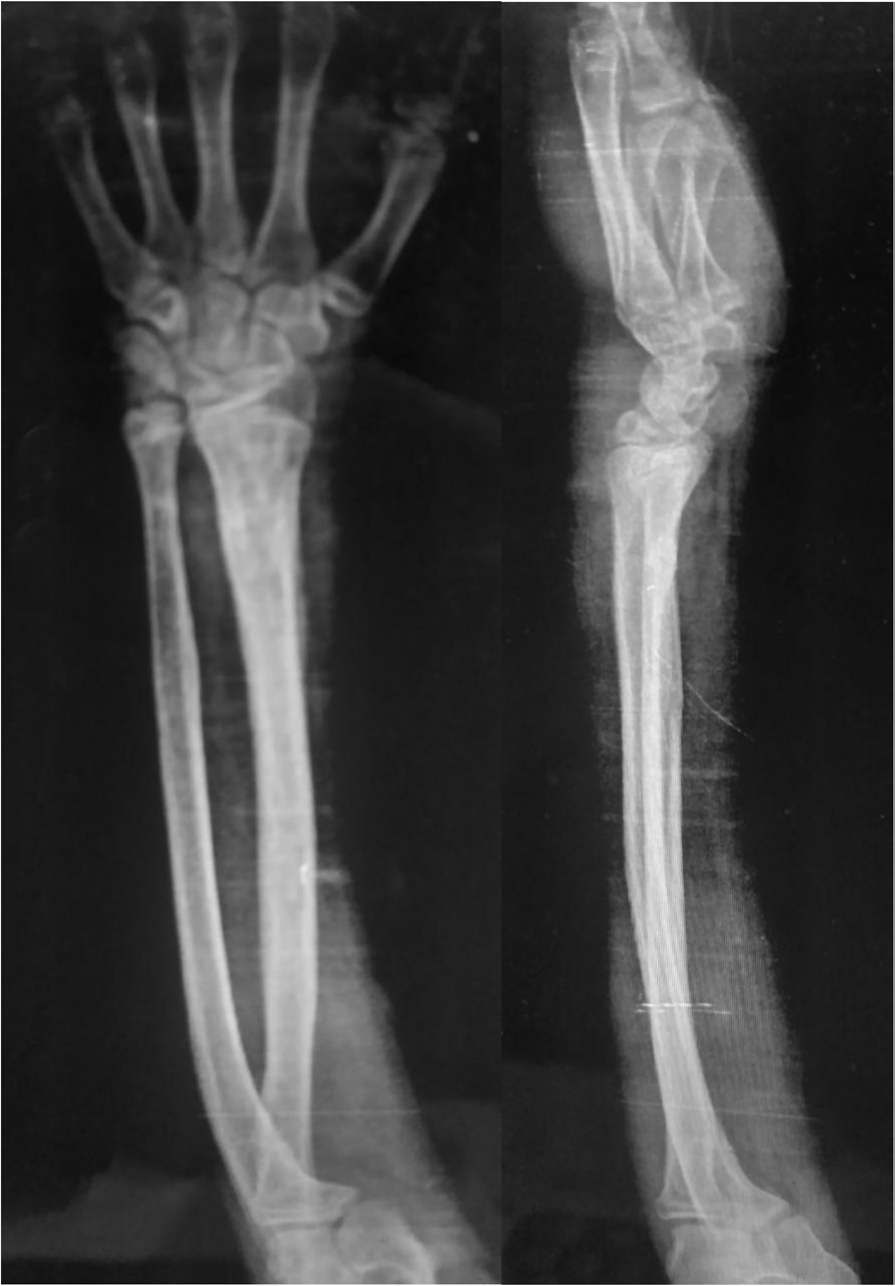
Anteroposterior and lateral X-ray views show the final follow-up 1 year postoperatively
